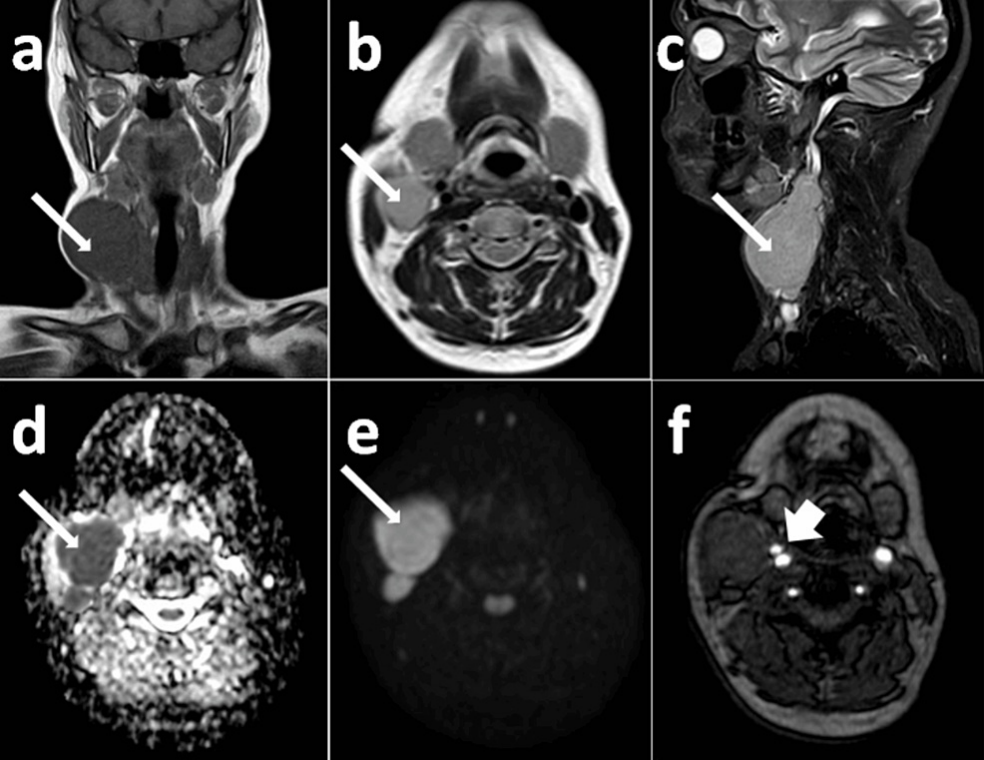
MRI images of the neck demonstrating a lesion in the right carotid space. (a) MRI T1-weighted image coronal section showing a well-defined T1 hypointense lesion (long white arrow) epicentered in the right carotid space. (b) MRI T2-weighted image axial section showing a well-defined T2 hyperintense to muscle lesion (long white arrow) epicentered in the right carotid space. (c) MRI STIR image sagittal section showing a well-defined hyperintense to muscle lesion (long white arrow) epicentered in the right carotid space. (d) ADC map of the neck shows low ADC levels involving the lesion (long white arrow) epicentered in the right carotid space. (e) The lesion shows corresponding diffusion restriction (long white arrow). (f) MRA axial section showing medial displacement of right internal and external carotid vessels (short white arrow). STIR: short tau inversion recovery; ADC: apparent diffusion coefficient; MRA: magnetic resonance angiography
